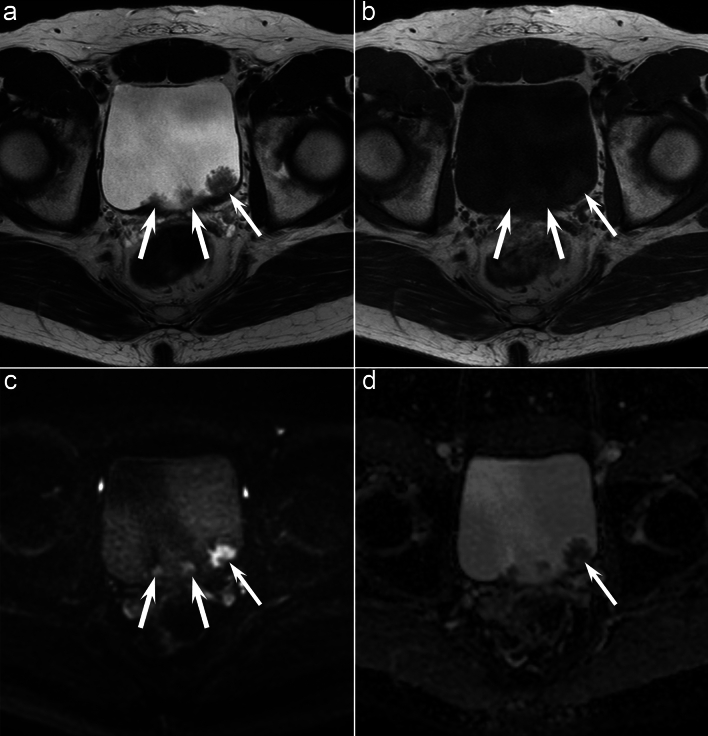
A 54-year-old man with UC. Axial T2-weighted image a and axial T1-weighted image b showing multiple pedunculated bladder masses (arrows) with irregular margins. c Axial diffusion-weighted image shows masses that is hyperintense (arrows). d Axial ADC map shows mildly restricted diffusion, with intermediate ADC values (1.376 × 10^−3^ mm^2^/s) (arrow)
